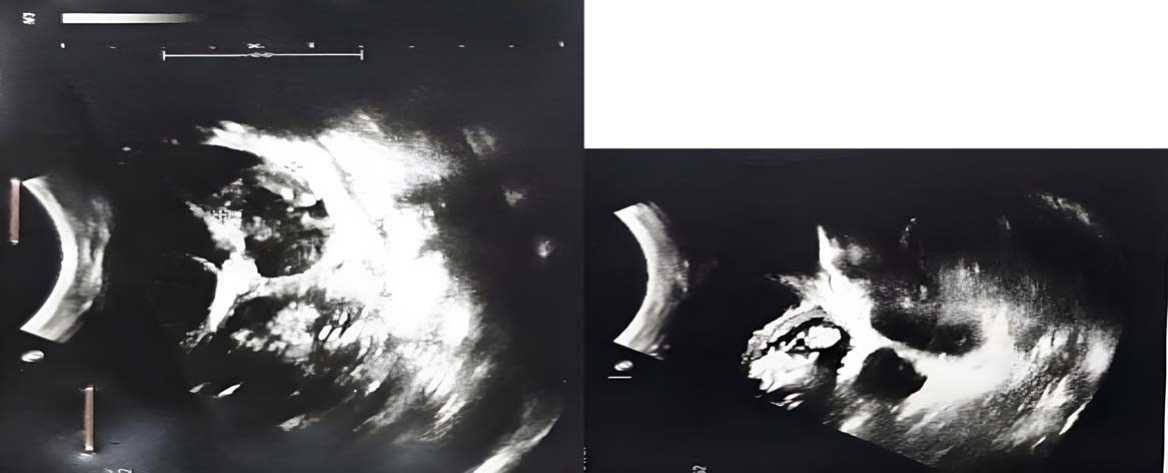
Abdominal ultrasound revealed a small bowel dilated about 24 mm with retrograde peristalsis and no ascites or free fluid in the peritoneal cavity.
